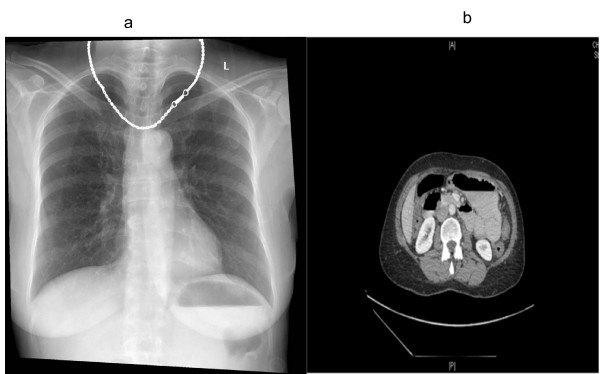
Imaging. a) Erect chest radiograph showing an air-fluid level within a dilated stomach. Lung fields were clear. There is no air under the diaphragm. b) Contrasted abdominal computed tomography showed possible stricture at the site of the gastrojejunostomy.
